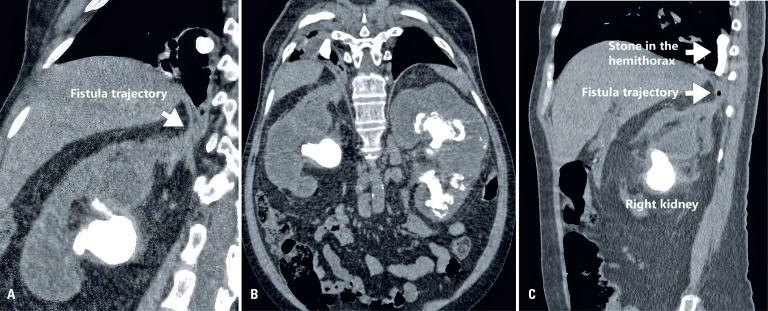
A) Computed tomography (CT) scan of the abdomen shows bilateral coralliform stones with pyelocalycial dilation. B) CT scan of the abdomen shows the fistulous tract and calculus in the right hemithorax. C) Chest CT scan shows calculus in the right kidney, the fistulous tract and the calculus into the right hemithorax
